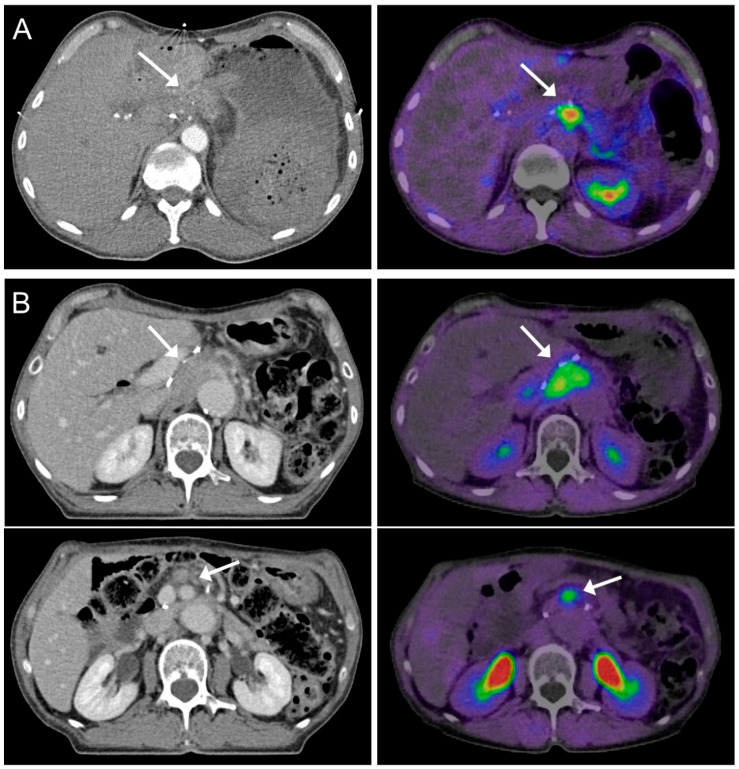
Precision of fibroblast activation protein inhibitor positron emission tomography/computed tomography (FAPI-PET/CT) compared to CT. (A) Imaging of a 77-year old patient suffering from locally recurrent pancreatic ductal adenocarcinoma (PDAC). Based on CT imaging on the left, the tumor (white arrow) can hardly be differentiated from the surrounding tissue. It can be located relatively precisely in the fused slice of the according FAPI-PET/CT scan on the right. (B) Imaging of a 71-year old nodal positive locally recurrent PDAC patient. The main tumor (white arrow) can be defined adequately in CT imaging as well as in FAPI-PET/CT imaging (upper right). In the lower left, the white arrow marks a lymph node that can easily be overlooked without FAPI-PET/CT information (lower right). In the present study, none of the six radiation oncologists contoured this lymph node as part of the gross tumor volume (GTV).
